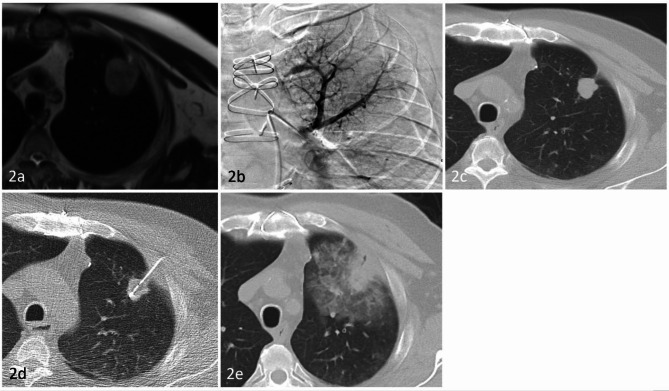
A 66 Y/O male suffered from LUL NSCLC. MR image before TPCE (2a) showed a LUL mass (4.3 cm). First TPCE was performed (2b) smoothly. After 3 sessions of TPCE. CT image (2c) showed size decreasing (4.3 to 2.3 cm) of the index lesion. MWA was performed subsequently (2d). CT image (2e) after MWA. ITS - Index tumor size. LUL- Left upper lung. NSCLC - Non-small cell lung cancer. MWA - Microwave ablation. TPCE - Transpulmonary chemoembolization.
